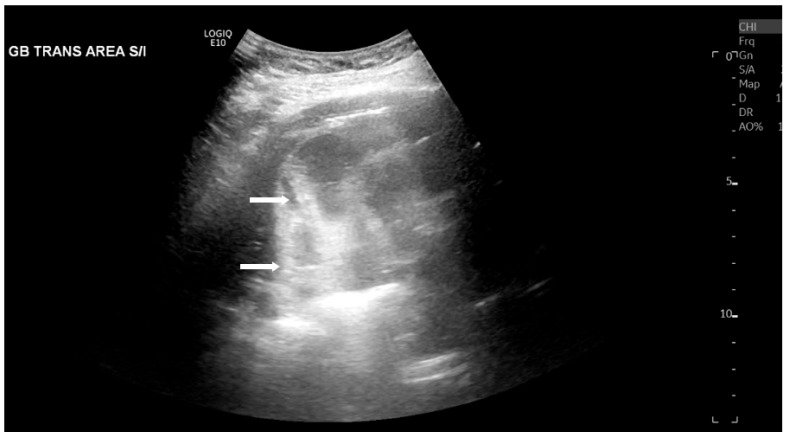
Right upper quadrant ultrasound of a 63-year-old male diabetic patient with emphysematous cholecystitis. Posterior dirty shadowing (white arrows) due to gas within the gallbladder wall were demonstrated.
